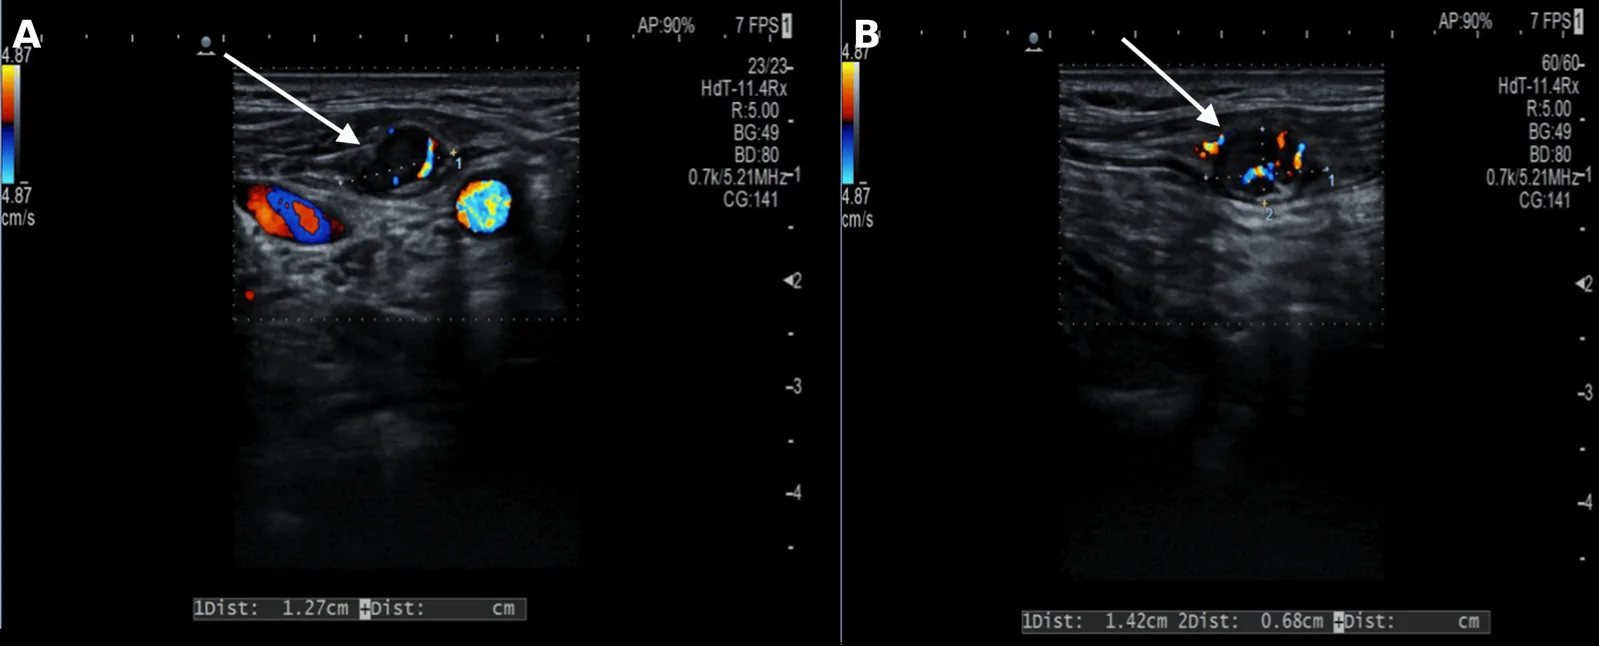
Targeted ultrasonographic reassessment of the autotransplantation site following localization by ¹⁸F-choline PET/CT. (A) Transverse color Doppler image demonstrating a well-circumscribed hypoechoic hypervascular lesion within the right sternocleidomastoid muscle. (B) Longitudinal view of the same lesion measuring 1.42 × 0.68 cm, corresponding to the hyperfunctioning lesion identified on ¹⁸F-choline PET/CT.
